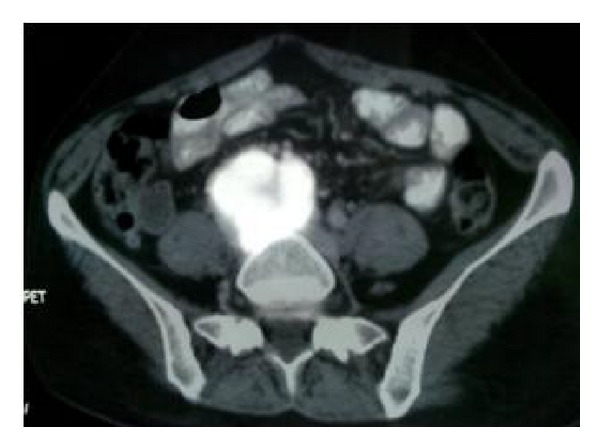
PET CT axial cut showing intense tracer uptake in the pelvic mass abutting the right psoas muscle.
